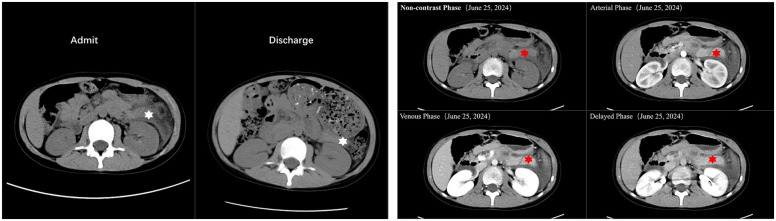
Contrast-enhanced abdominal CT scan of the patient confirmed acute pancreatitis. The pancreas (asterisk) enhances completely and was mildly enlarged without pancreatic duct dilatation or parenchymal necrosis. Marked inflammatory infiltrates and fluid collections are present around the pancreatic body and tail.
